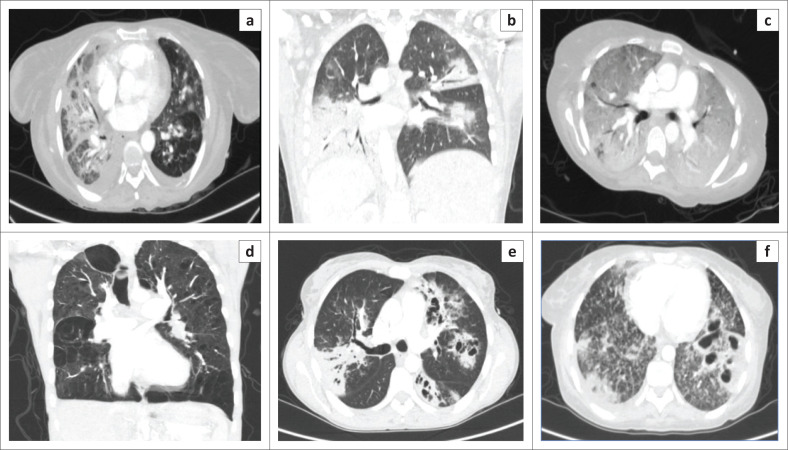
Axial (a, c, e, f) and coronal (b, d) CT slices of incidental parenchymal findings in patients without pulmonary embolism. (a–c) Extensive consolidations in three different patients, (d) diffuse emphysema, (e) cavitary multi-lobar pneumonia, and (f) left lower lobe lung cavitation with bilateral background tree-in-bud nodules in a patient with pulmonary tuberculosis.
